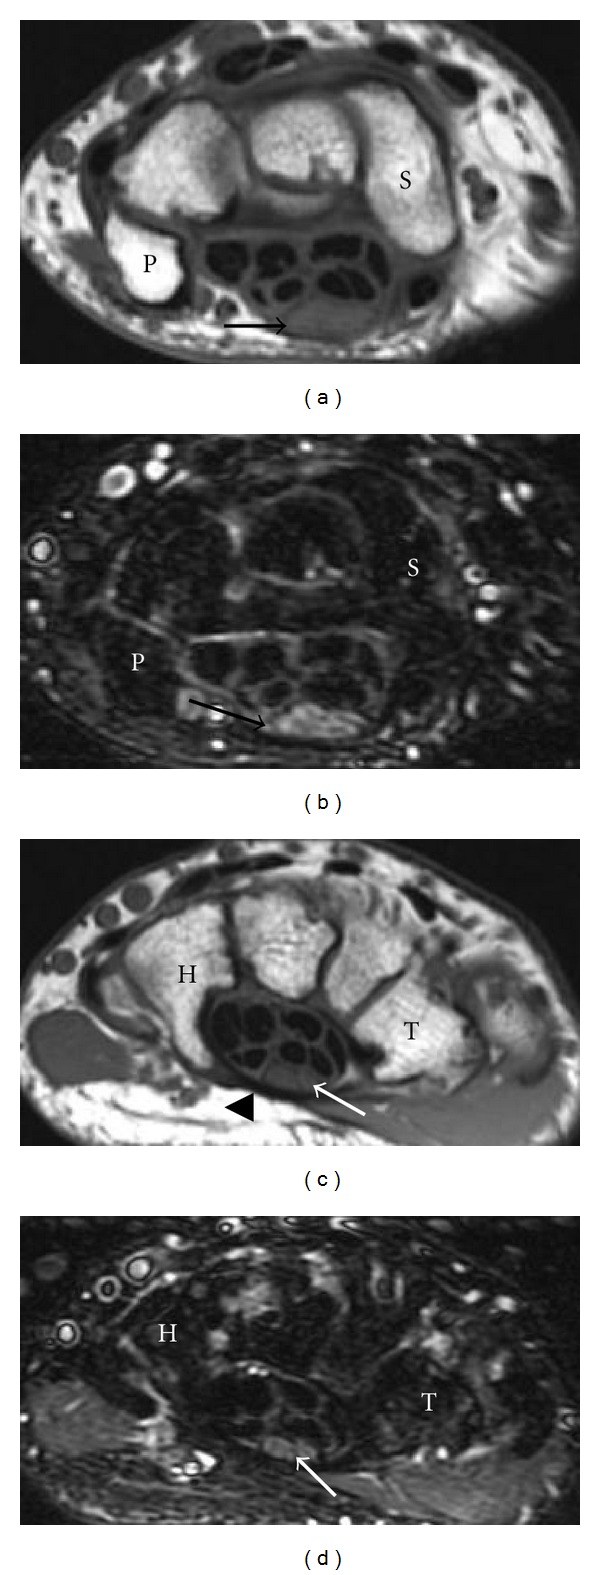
A 48-year-old patient with surgically proven right carpal tunnel syndrome. There is thickening and increased signal intensity of the median nerve (black arrows) at the level of pisiform (P) and bowing of the flexor retinaculum (arrowhead) with a flattened median nerve (white arrows) at the level of hamate (H). (a) and (c) are T1-weighted images, and (b) and (d) are T2-weighted images with fat saturation.
